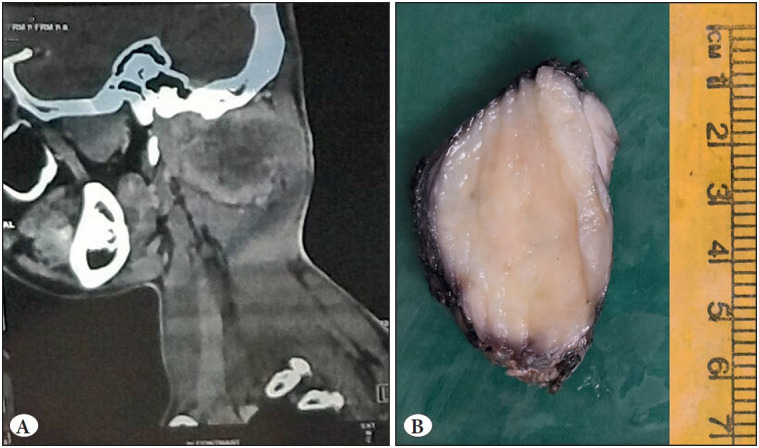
A) MRI revealing a lesion measuring 5.9 cm in the largest dimension, extending from the skull base superiorly up to the C2 vertebral level, abutting the occipital bone. B) Cut surface of the resected tumor showing a wellcircumscribed border with a greyA B white homogenous surface.
